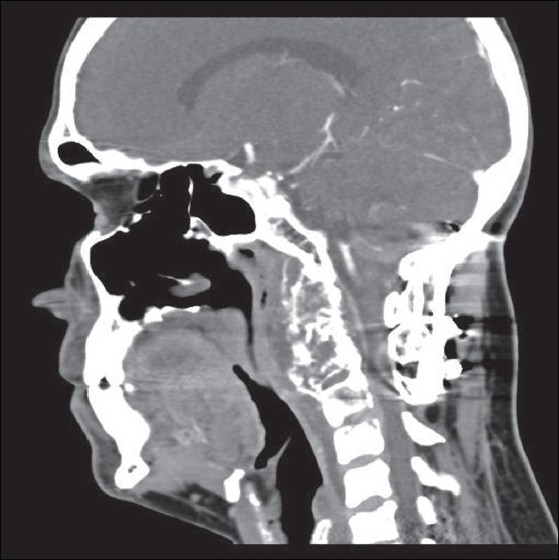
CT Scan (sagital view) showing the lesion
